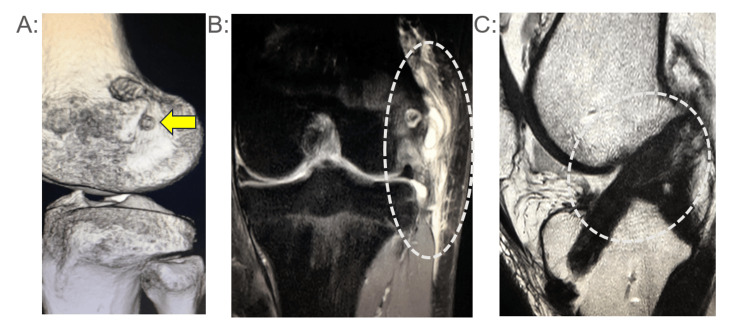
Case 2 (CT・MRI) (A) Postoperative CT image. The arrow marks the location of the bone tunnel created for the lateral extra-articular tenodesis (LET). (B) MRI at the time of reinjury (fat-suppressed sequence): evidence of damage is seen at the LET site. (C) MRI at the time of reinjury (T2-weighted sagittal view): the anterior cruciate ligament (ACL) remains intact
